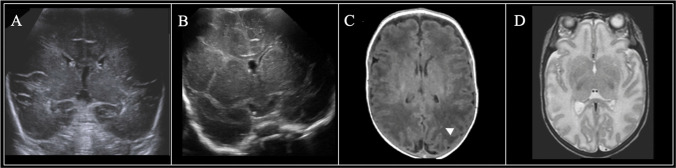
Neuroimaging findings in an infant with no evidence of white matter injury on head ultrasound. The male infant was born at 28 weeks and 5 days. Sequential imaging demonstrated (A) normal 7-DOL coronal-plane head ultrasound (Miller score 0); (B) normal 30-DOL coronal-plane head ultrasound (Miller score 0); and (C) term-equivalent MRI with a punctate focus of increased signal intensity in the white matter on T1-weighted imaging (*white arrowhead*, Martinez-Biarge score 1a). D Axial T2-weighted imaging was normal
